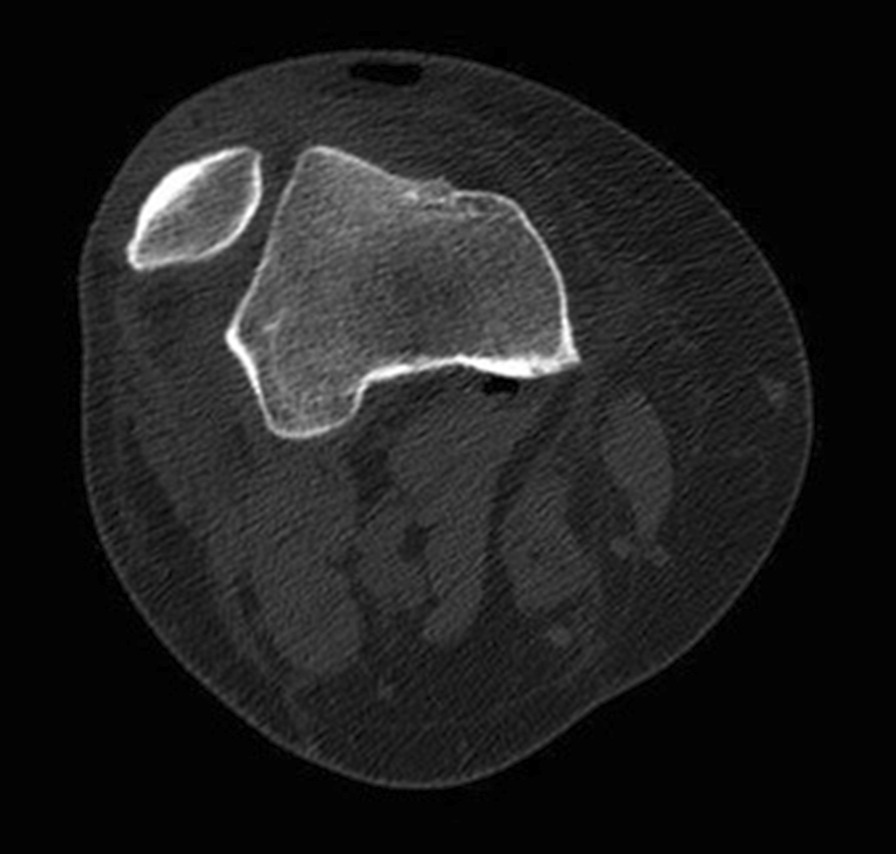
Axial view computed tomography demonstrating patellar dislocation
